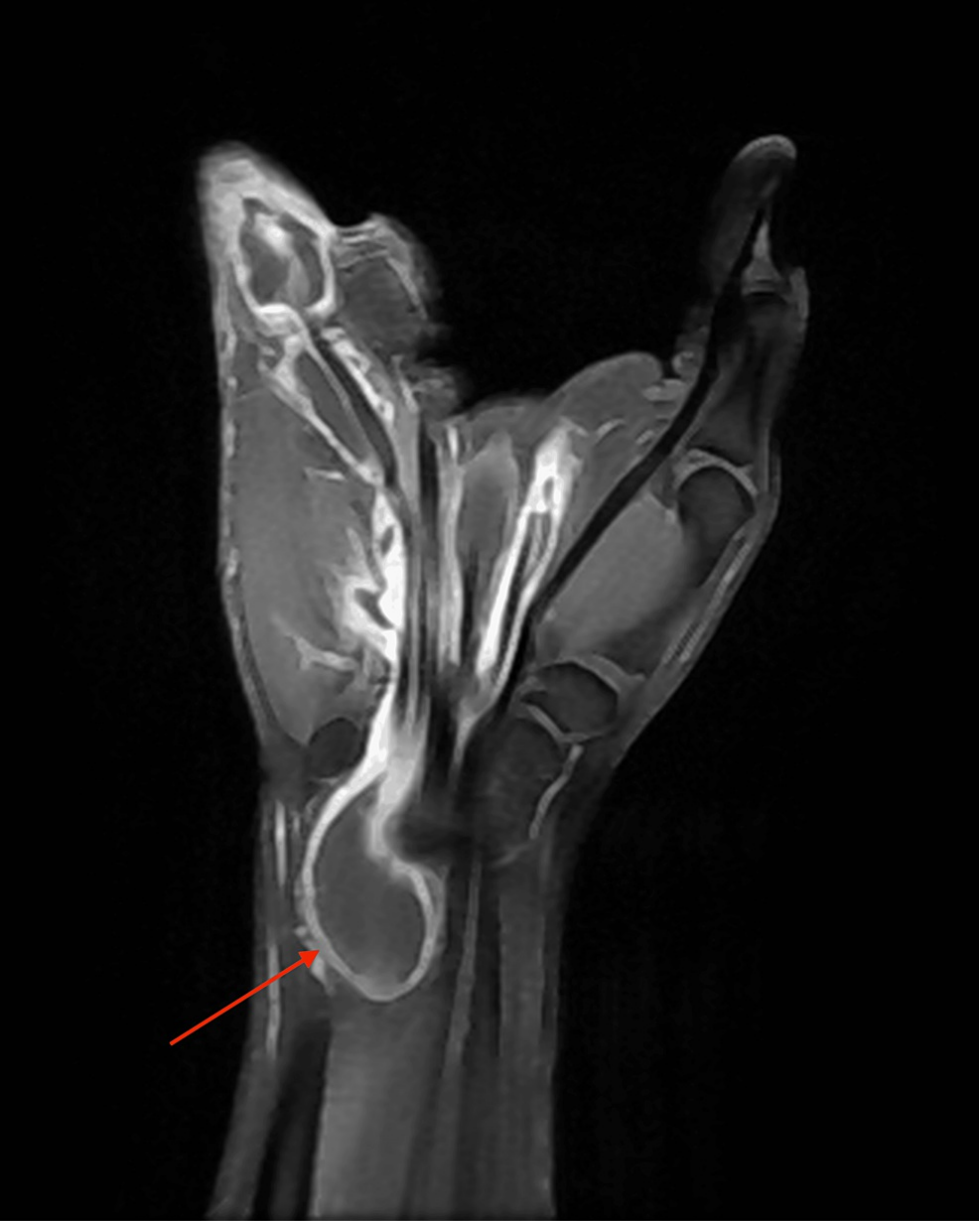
MRI of the left hand in coronal view showing diffuse thickening and enhancement of the tendon sheath of the flexor digitorum superficialis
